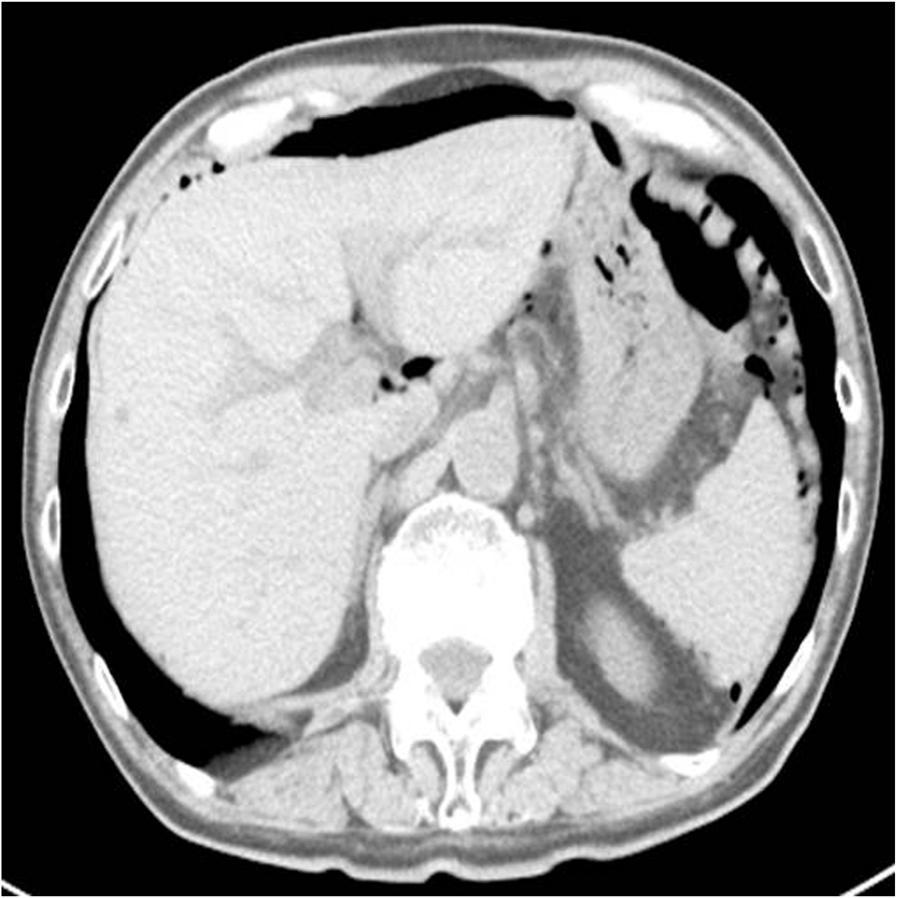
Abdominal CT showing an amount of free gas without intra-abdominal fluid collection in the upper abdominal cavity
